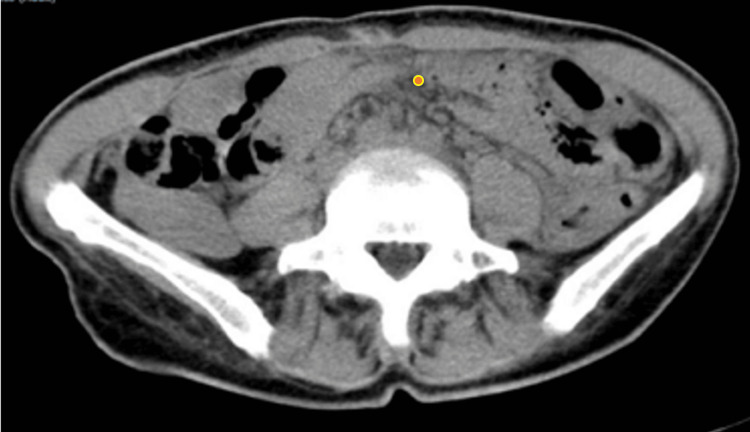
Pelvic CT (image 2 of 2) Axial CT image showing densification of all intraperitoneal adipose planes (●), suggestive of inflammatory fat stranding.
